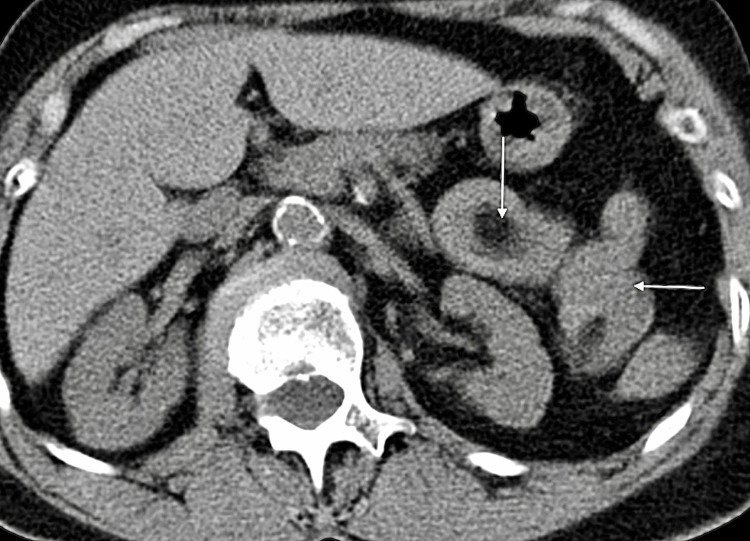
Axial non-contrast CT scan to the upper abdomen showing the “target sign” as evidenced by alternating varying mural densities within the proximal jejunum (vertical arrow) There is also focal mural thickening at the antimesenteric border of the jejunum (horizontal arrow).
